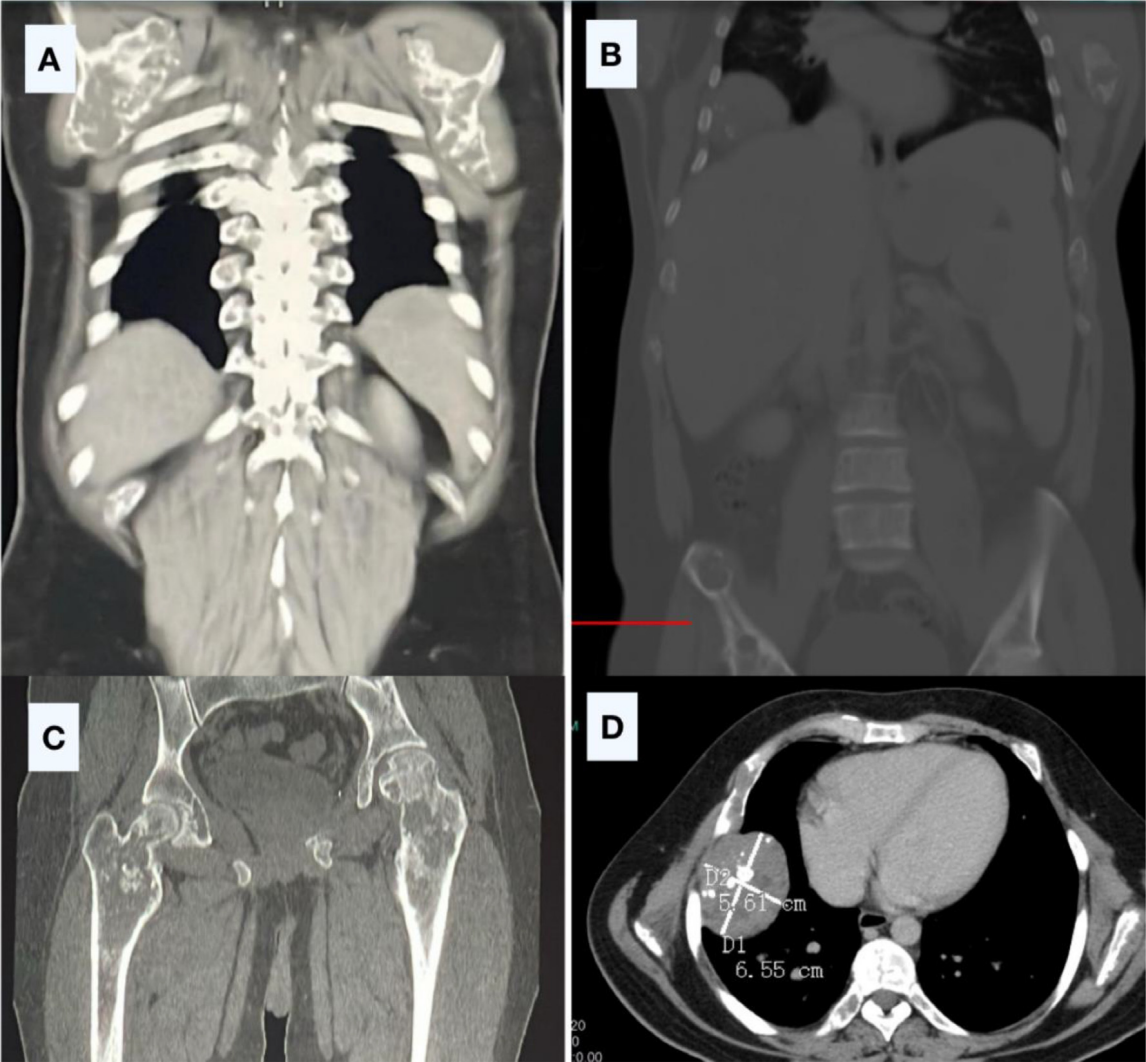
CT Chest, Abdomen, and Pelvis Findings: Multiple pulmonary nodules, concerning for metastases. Expansile lytic lesions with soft tissue components involving both scapulae (A, soft tissue window). Expansile lytic lesions in the pelvic bones, ribs, and scapulae (B, E, bone window). Multiple lytic lesions in both femur and the visualized pelvic bones (C). A large subpleural soft tissue lesion involving the right sixth rib, suspicious for malignant degeneration of an enchondroma (D).
